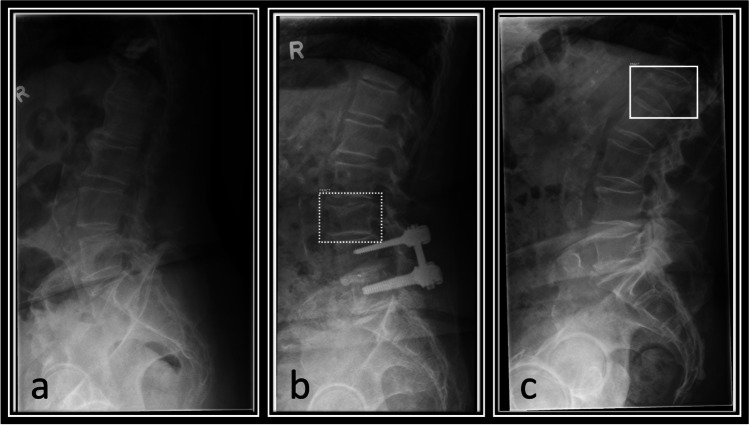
A–c From left to right with markings by the AI software: lateral radiographs of the lumbar spine from different patients. Overlay of images returned by the AI showing no regions of interest (a), a dashed-line bounding box (b) where a fracture is deemed possible and a through line bounding box (c) where a fracture is deemed likely. b and c show true positive results by the AI
